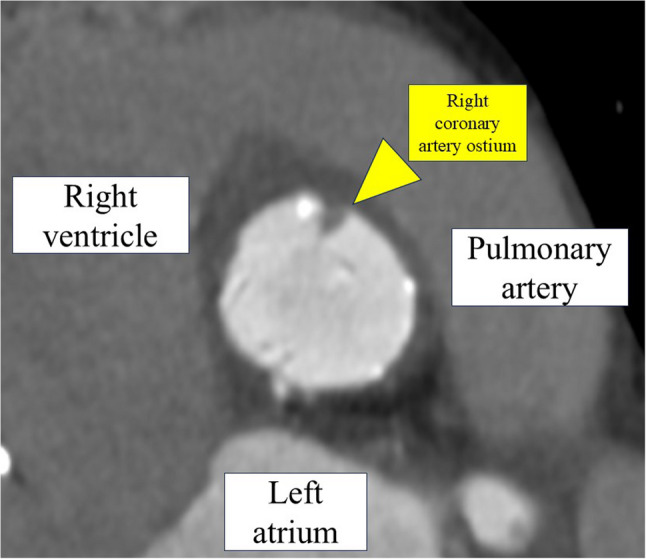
Contrast-enhanced computed tomography of the right coronary artery ostium. Contrast-enhanced computed tomography demonstrates a low-attenuation lesion at the ostium of the right coronary artery (yellow arrowhead). The anatomical relationships, including the right ventricle, pulmonary artery, and left atrium, are labeled for reference
